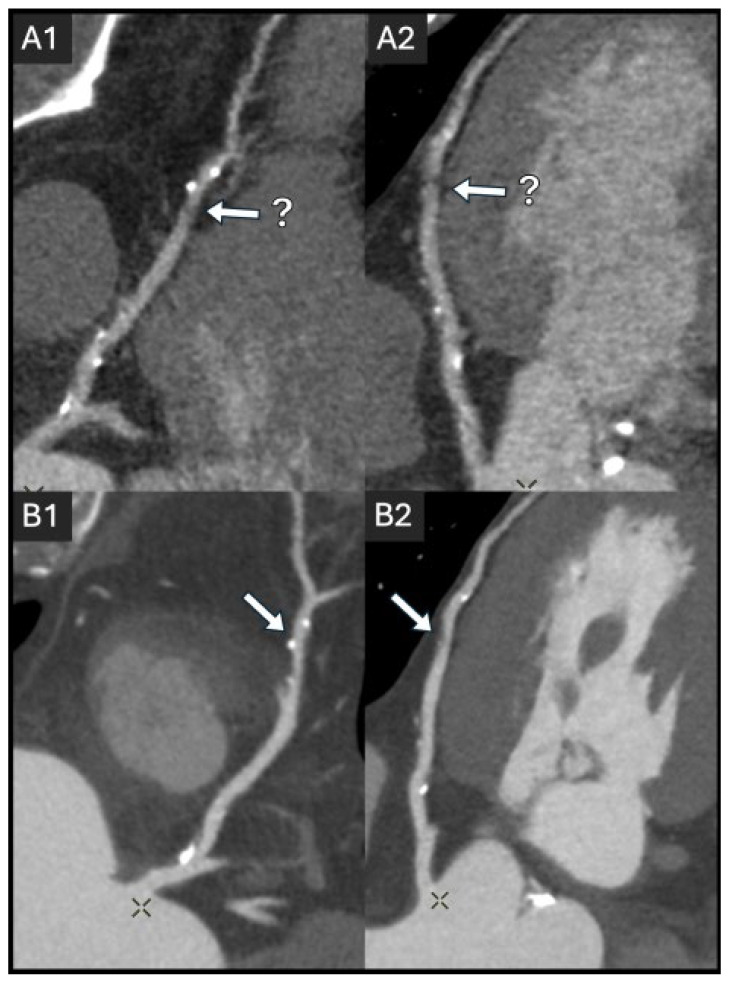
An example of the effect of suboptimal iodine delivery in a 63-year-old patient with endocarditis and severe aortic stenosis, undergoing coronary CT angiography (CCTA) to rule out coronary stenosis. The initial scan, performed at 87 bpm via an 18 G peripherally inserted central catheter (PICC), resulted in a limited maximum flow rate (50 mL, approximately 3 mL/s). Due to insufficient lumen attenuation and limited diagnostic accuracy (A1,A2), this scan did not allow for ruling out a stenosis of 50% or more, with a plaque of undetermined diameter stenosis (white arrow with a question mark). While the PICC model was designed to handle up to 5 mL/s flow, several factors can influence whether this flow is achievable, including the catheter length, contrast medium (CM) viscosity, and injection pressure. A repeat CCTA, following proper venous access (18 G IV catheter in the antecubital fossa) and beta-blocker preparation (72 bpm), achieved optimal image quality using 50 mL of CM at 5 mL/s with motion correction (B1,B2) and showed a <50% stenosis (white arrow). The images are displayed in a curved MPR view at 0° and 90° rotation, respectively. The CT protocol was exactly the same for both acquisitions: tube voltage, 100 kVp; tube current, 900 mA; and gantry revolution time, 0.23 s. The difference in noise between (A1,A2) and (B1,B2) is due to the lower heart rate in the repeat scan. At a lower heart rate, more projections (or data samples) contribute to the reconstruction of a single slice, resulting in lower noise despite identical acquisition parameters.
